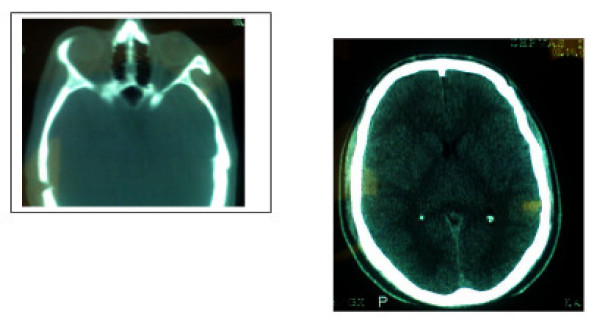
Pre-treatment/post-treatment CT scan.
